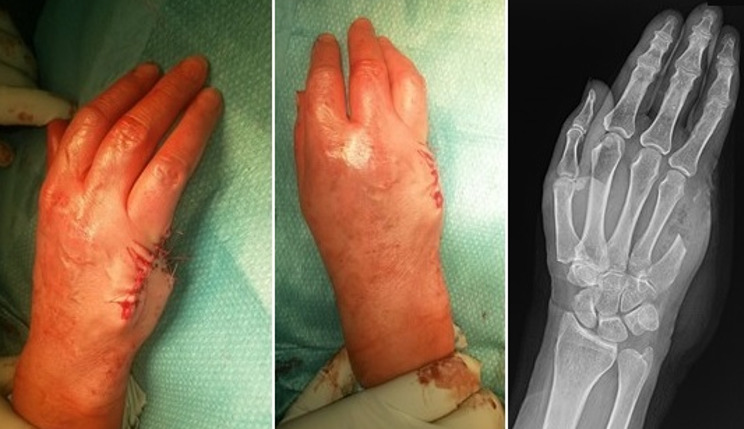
Surgical and radiographic result immediately after surgery
